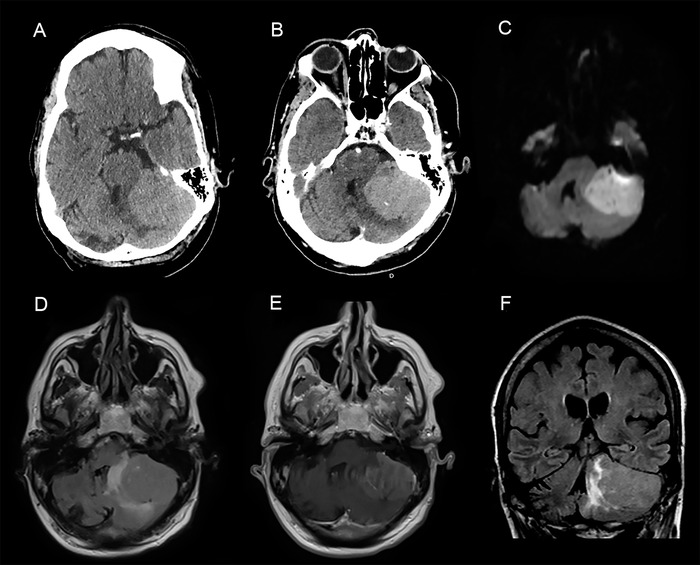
Axial (A) noncontrast and (B) postcontrast head computed tomography images demonstrate a hyperdense, enhancing extra-axial mass lesion of the left cerebellopontine angle. (C, D, E) Axial and (F) coronal brain magnetic resonance images, with and without contrast, demonstrate an enhancing extra-axial posterior fossa mass in the left cerebellopontine angle, exhibiting diffusion restriction (C: diffusion-weighted imaging [DWI]), perilesional edema (F: fluid attenuated inversion recovery [FLAIR]), mass effect, and rightward deviation of the fourth ventricle (F: FLAIR).
